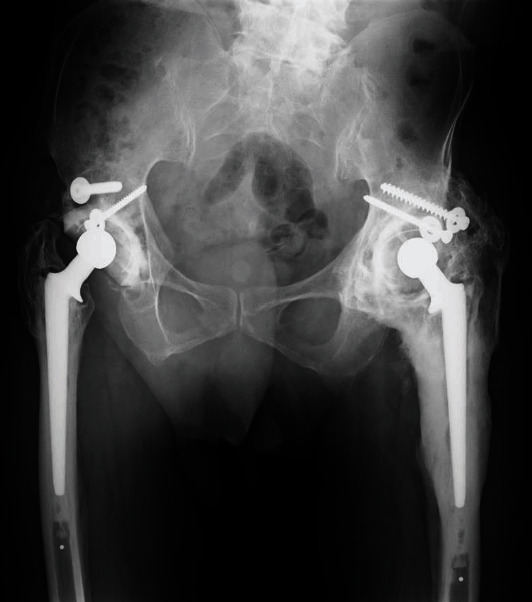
Plain radiograph obtained at the initial visit of a 78-year-old man, showing severe heterotopic ossification around the left hip joint.
